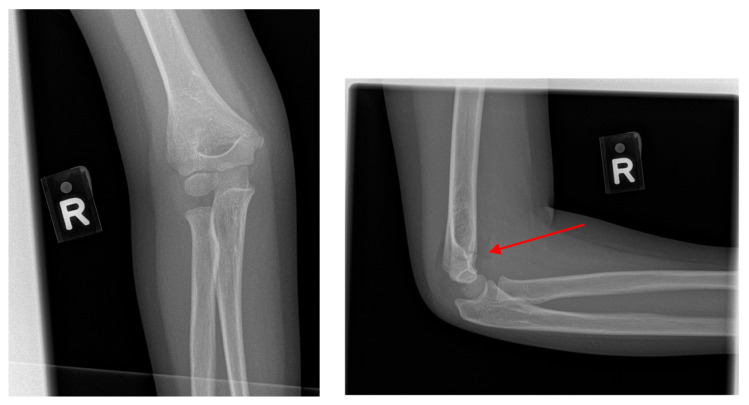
Example of a false negative case where an undisplaced supracondylar fracture of the right humerus (indicated by the post-processed red arrow on the lateral elbow radiograph) was misinterpreted by ChatGPT 4o as a ‘normal’ study.
